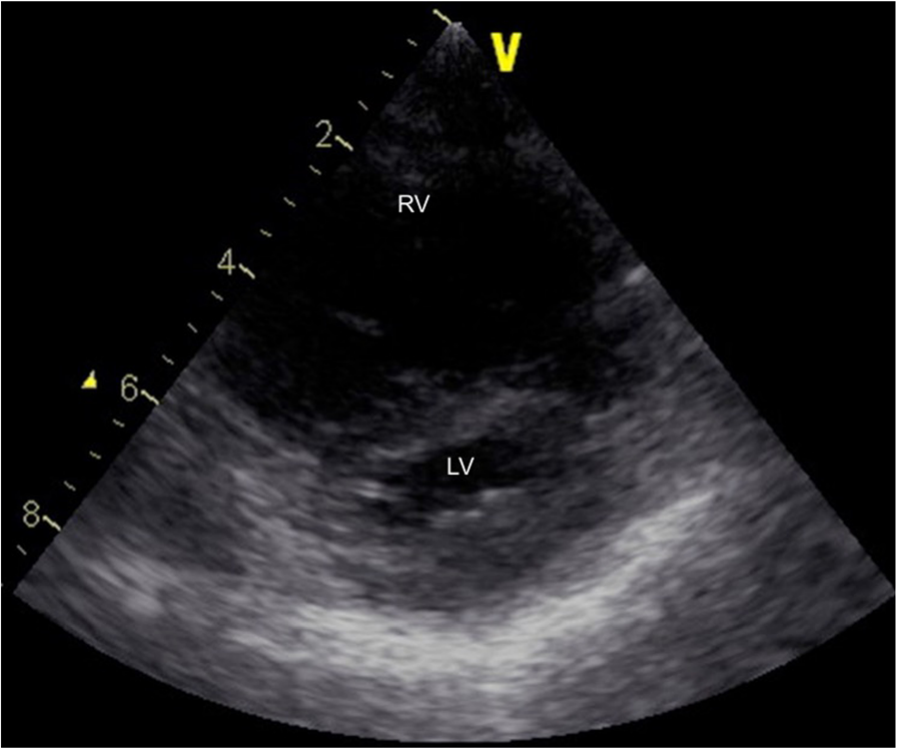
Echocardiogram in the short-axis view showing displacement of the ventricular septal wall due to the enlarged right-side heart. RV, right ventricle; LV, left ventricle
